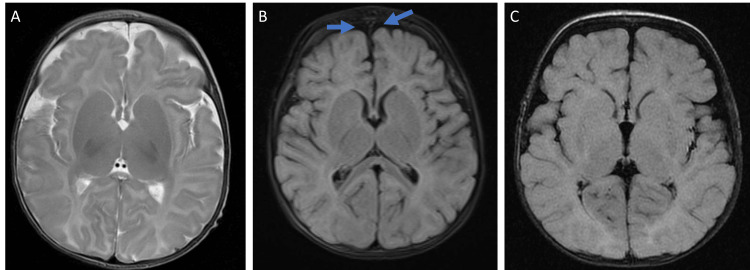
Brain MRI of the three reported ARV1 cases. (A) Unremarkable axial T2-weighted brain MRI. (B) T2-weighted fat-stranding brain MRI showed slightly enlarged bifrontal subarachnoid spaces (arrow). (C) Unremarkable axial FLAIR-weighted brain MRI.
